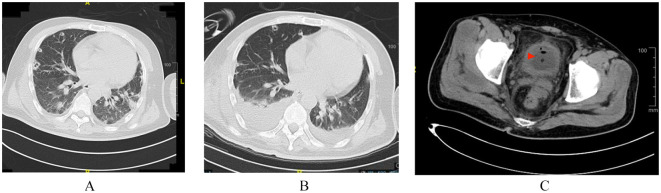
Bacteria migrate and spread into the lungs; Chest CT (A) April 18 (B) May 11; Formation of rectal bladder abscess. Pelvic CT. (C) April 19.
